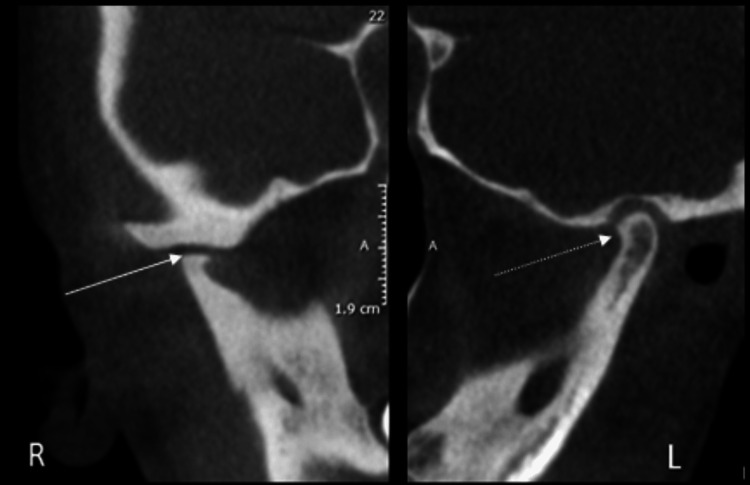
CBCT corrected sagittal sections in maximum intercuspation position showing right and left TMJ with severe degenerative changes on the right side (solid white arrow) and moderate flattening on the left side (dashed white arrow) CBCT: Cone Beam Computed Tomography, TMJ: temporomandibular joint
